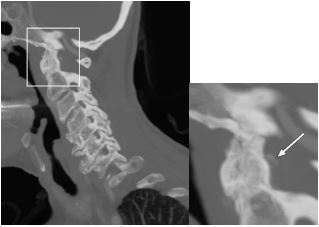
Computed tomography angiogram of the head and neck, sagittal view, showing calcification of the periodontoid ligament (arrow) surrounding the odontoid process.
